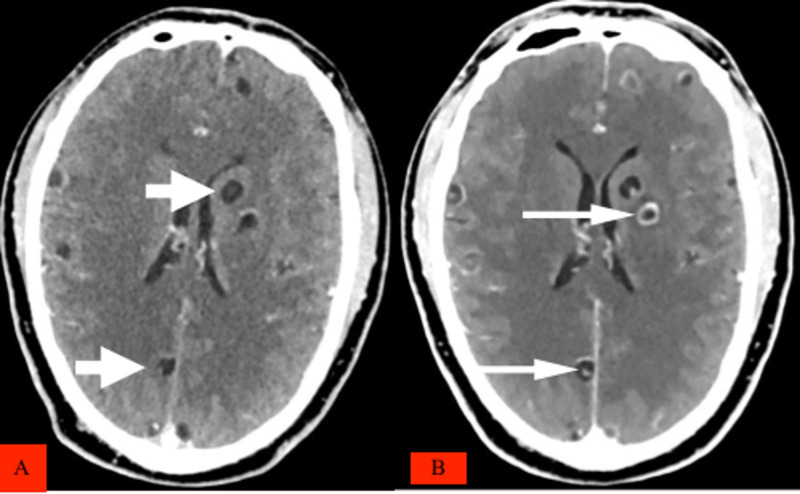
Axial IV contrasted brain CT showing (A) numerous cystic hypodense lesions pointed by short arrows, and (B) fewer calcified cystic lesion six weeks after treatment, some with a dot sign pointed by long arrows.
